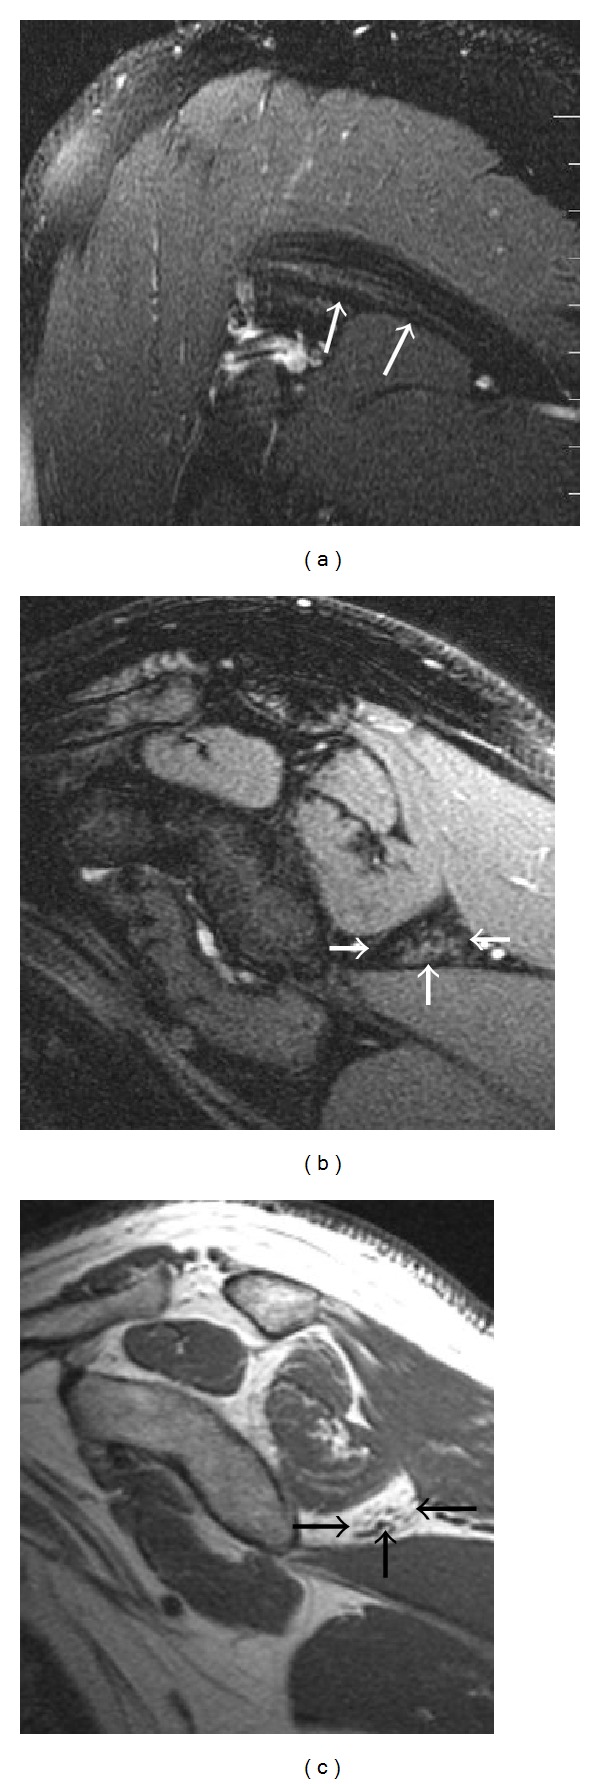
A 46-year-old patient with right shoulder pain and clinical and EMG evidence of quadrilateral space syndrome. Oblique coronal (a), oblique sagittal (b) T2 fat-saturated, and oblique sagittal T1-weighted (c) images demonstrate severe fatty atrophy of the teres minor muscle (arrows).
